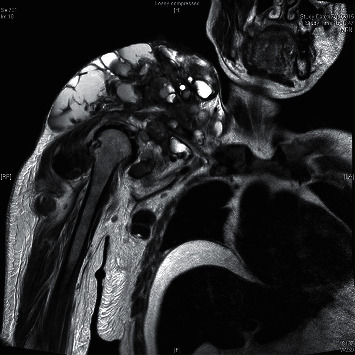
Magnetic resonance imaging (T2-weighted) of the right shoulder.
